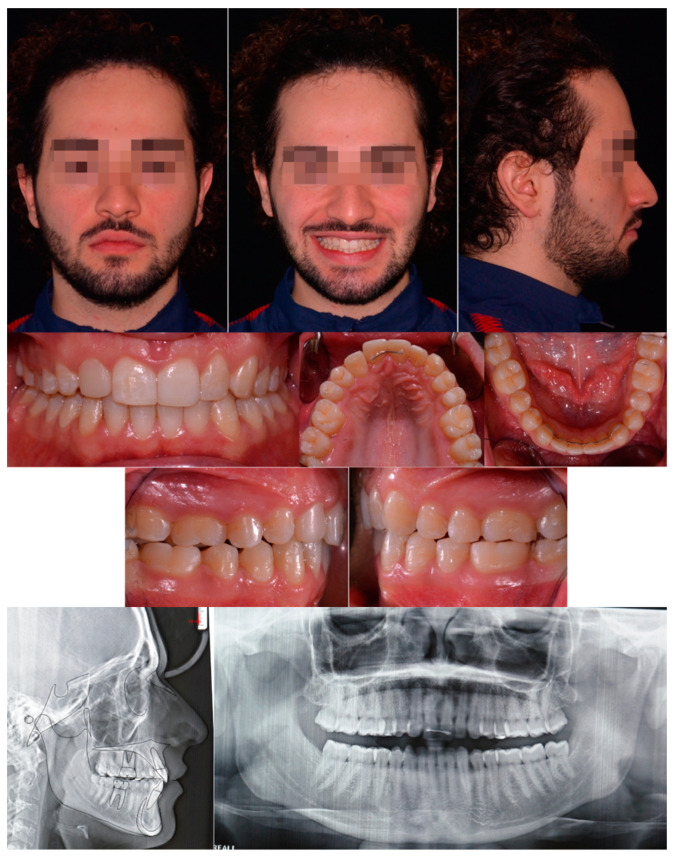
Final extra-, intra-oral, and radiographic records.
